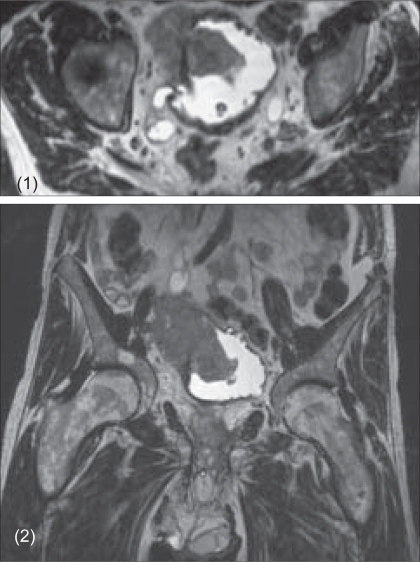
MRI of a pT4b TCC with consecutively dilated right ureter
